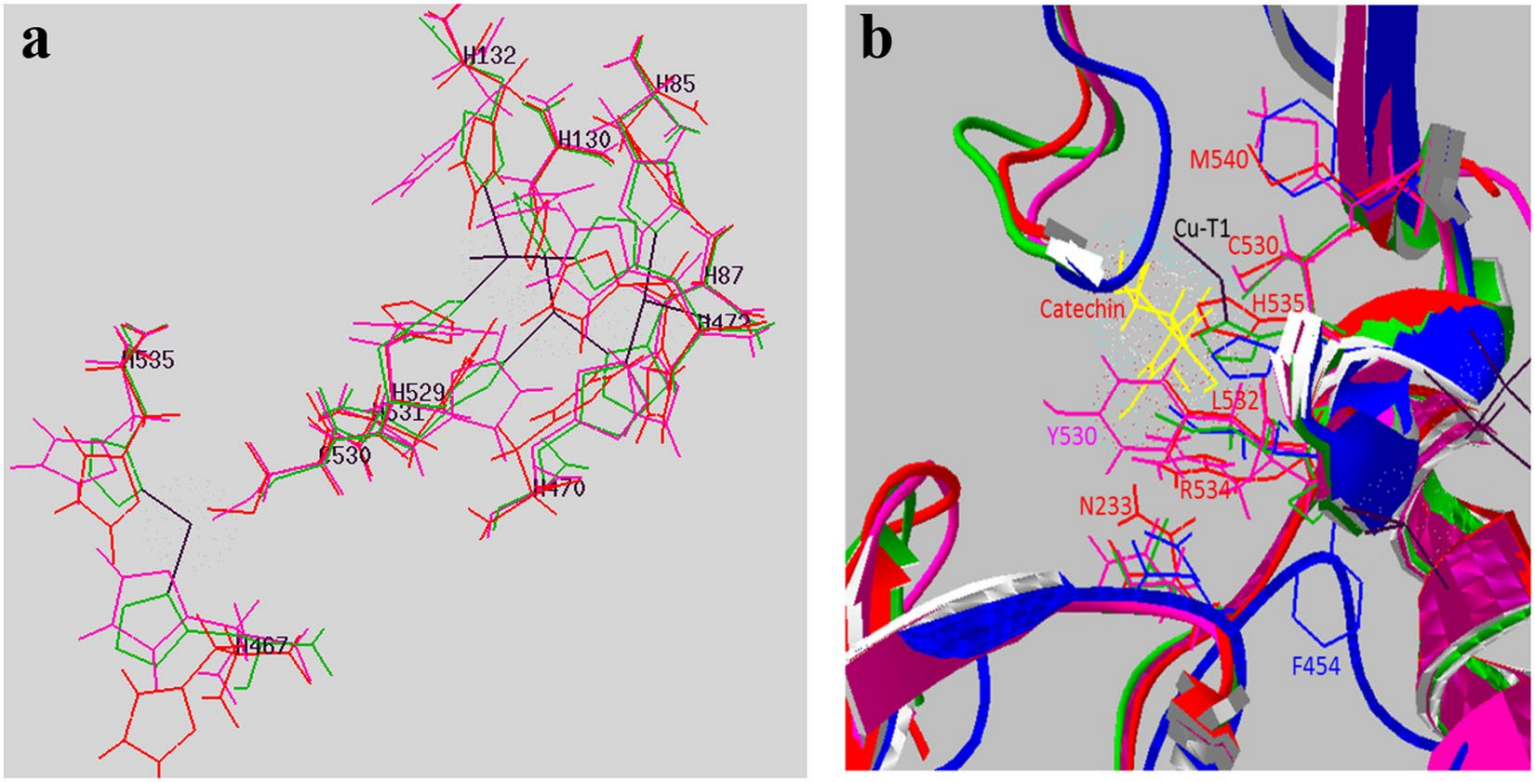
Fruit LMCOs modelled on the X-ray structure of ascorbate oxidase (Ao) along with the X-ray structure of fungal laccase from *Trametes trogii* (Tt) showing copper-interacting and critical catalytic residues. (a) Copper-interacting His and Cys residues. (b) Substrate-binding loops surrounding the active-site cleft and showing critical residues that interact with the substrate, involved in catalysis and redox potential. Red, cherry LMCO; pink, litchi LMCO; green, Ao; blue, Tt. Yellow, substrate (catechin); black lines, copper. Copper atom on the extreme left in both figures is T1. Residue numbering is based on cherry LMCO sequence and critical residues marked in Fig. S2b (multiple alignment) are depicted in their respective colors.
